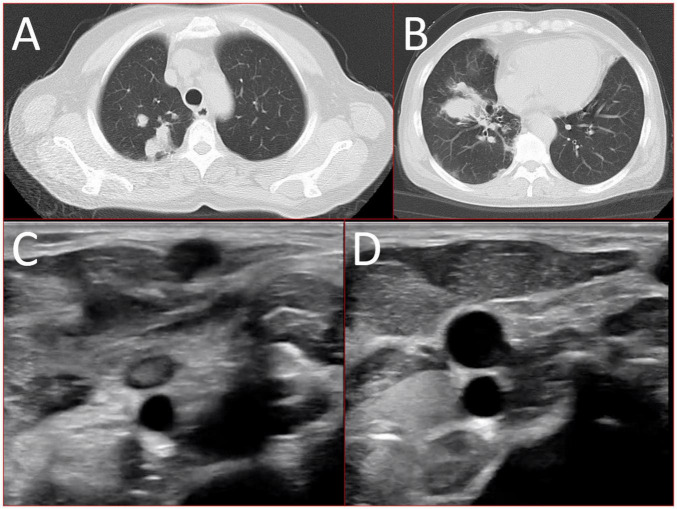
16-slice computerized tomography images of the chest at initial diagnosis. (A) Upper lobe; (B) lower lobe) and ultrasound images of the neck at initial diagnosis (C) inflammatory changes; (D) local effusion.
